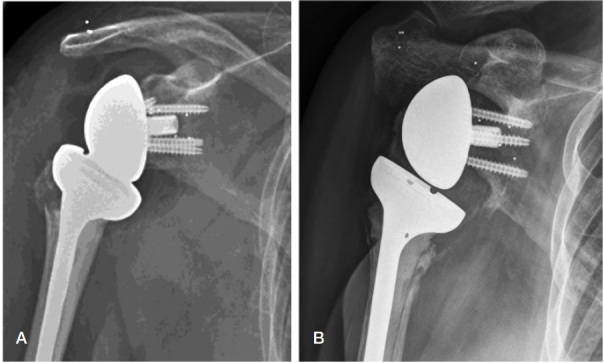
Radiograph of a patient operated with a reverse total shoulder arthroplasty postoperatively (A) and at 2-year follow-up (B), where “notching” grade 3 can be observed in the inferior scapular neck. RSA markers were implanted in the scapular neck, coracoid, and acromion.
